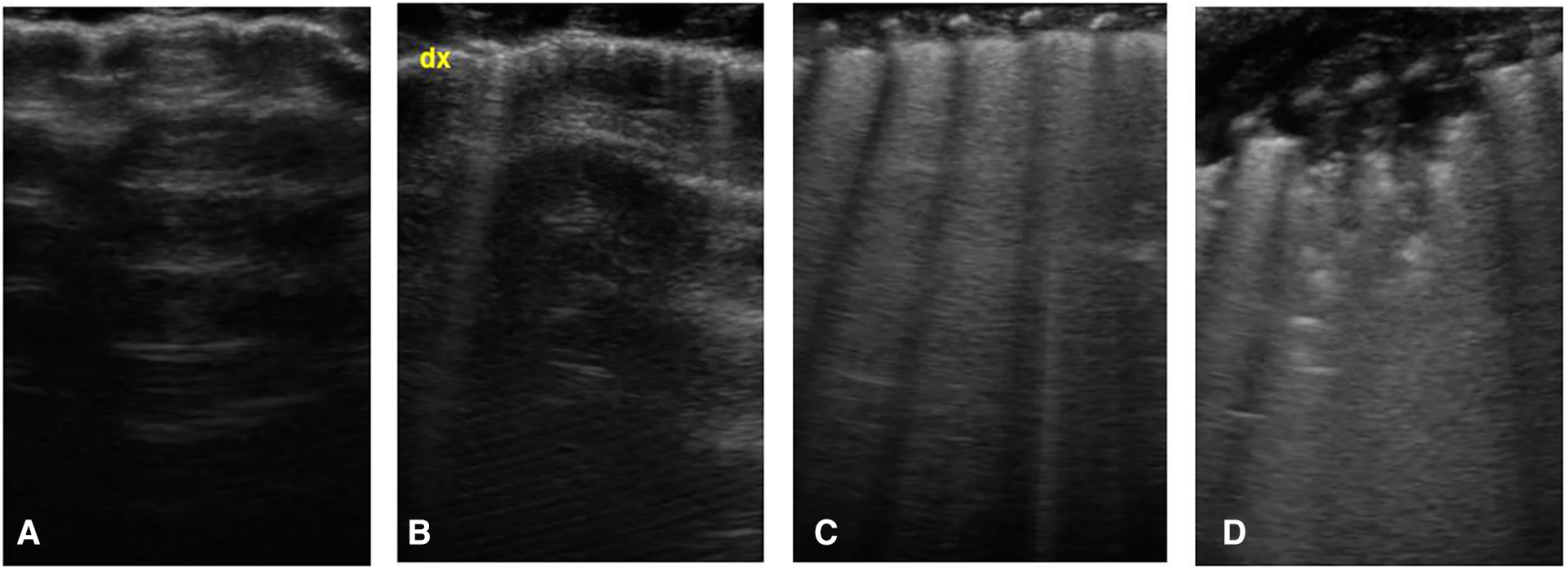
Lung ultrasound score, images obtained by longitudinal scan with a linear probe: (A) presence of only A-lines under the pleural line (normal pattern, score 0); (B) isolated vertical bright line, starting from pleural line and erasing the underlying A-lines, called B-line (score 1); (C) coalescent B-lines (score 2); (D) presence of consolidation (score 3).
